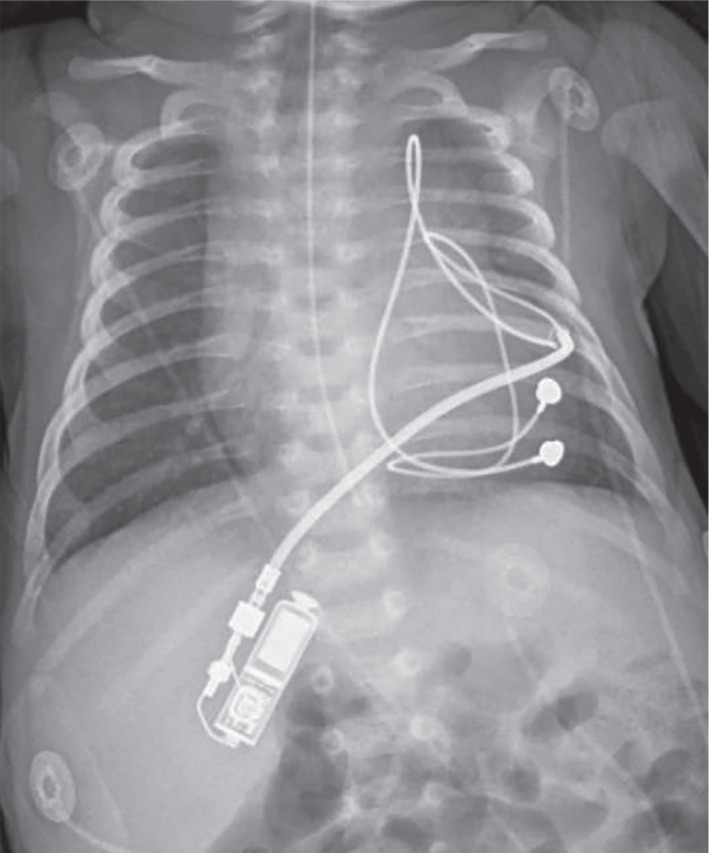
Chest radiography showing subxiphoid placement of the Micra™ implantable pulse generator connected to epicardial leads.
